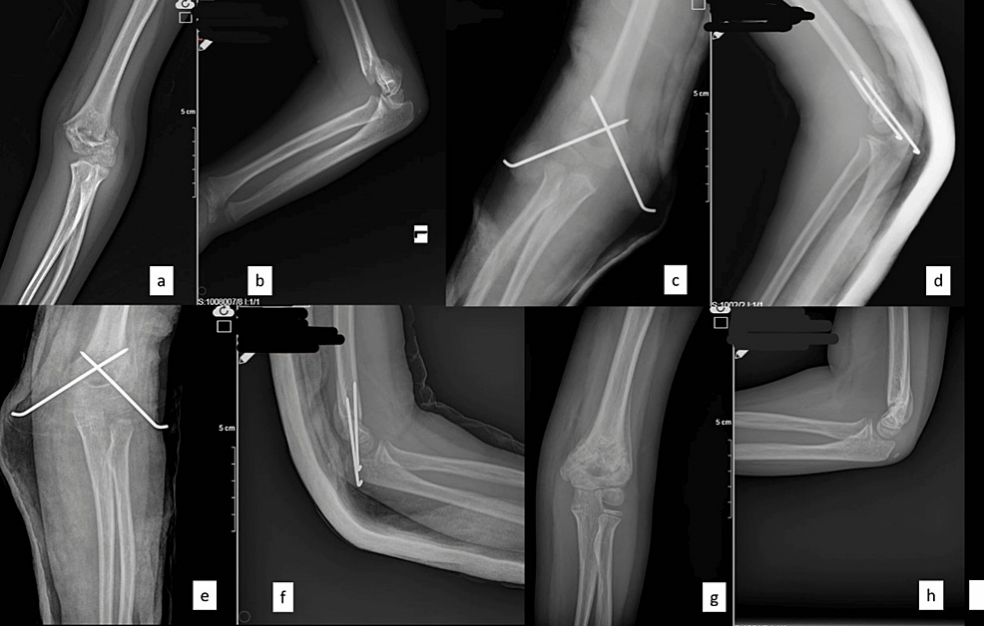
a) Preoperative elbow AP X-ray. b) Preoperative elbow lateral X-ray. c) Postoperative first-day elbow AP X-ray. d) Postoperative first-day elbow lateral X-ray. e) Postoperative third-week elbow AP X-ray. f) Postoperative third-week elbow lateral X-ray. g) Postoperative sixth-week elbow AP X-ray. h) Postoperative sixth-week elbow lateral X-ray
